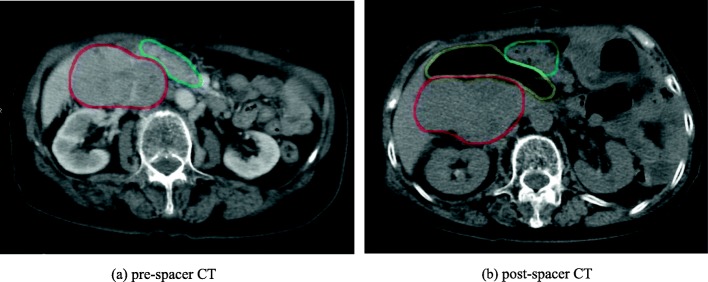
Example of pre-spacer CT (a) and post-spacer CT (b). The lines show the spacer (yellow), the tumor (red) and duodenum (blue)
